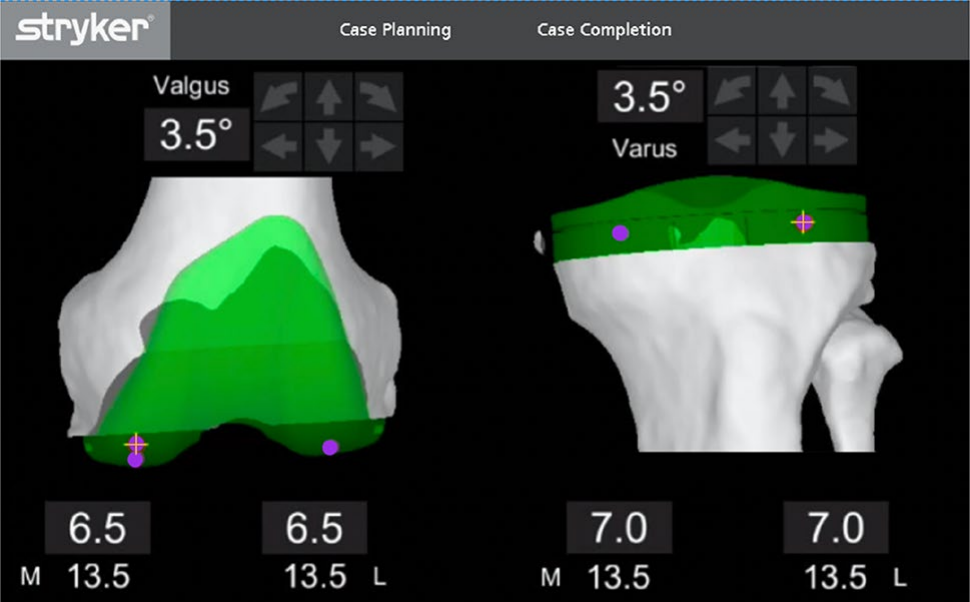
Computed tomographic determination of the arithmetic HKA angle. Determination of the computed tomographic arithmetic hip-knee-ankle angle (CT-aHKA) using the Mako software to determine the LDFA and MPTA. These are ascertained by application of matched resections (6.5 mm femur and 7.0 mm tibia) to the landmarks represented by the purple dots in this figure. The resultant coronal plane angle reported by the software (in this case 3.5° valgus for the femur and 3.5° varus for the tibia) is the precise deviation from the orthogonal axis. From these angles, we can infer the values of 86.5° for both the LDFA and MPTA and thereby calculate the CT-aHKA using the arithmetic method (CT-aHKA = MPTA − LDFA). *CT* computed tomographic, *aHKA* arithmetic hip-knee-ankle angle algorithm, *LDFA* lateral distal femoral angle, *MPTA* medial proximal tibial angle
